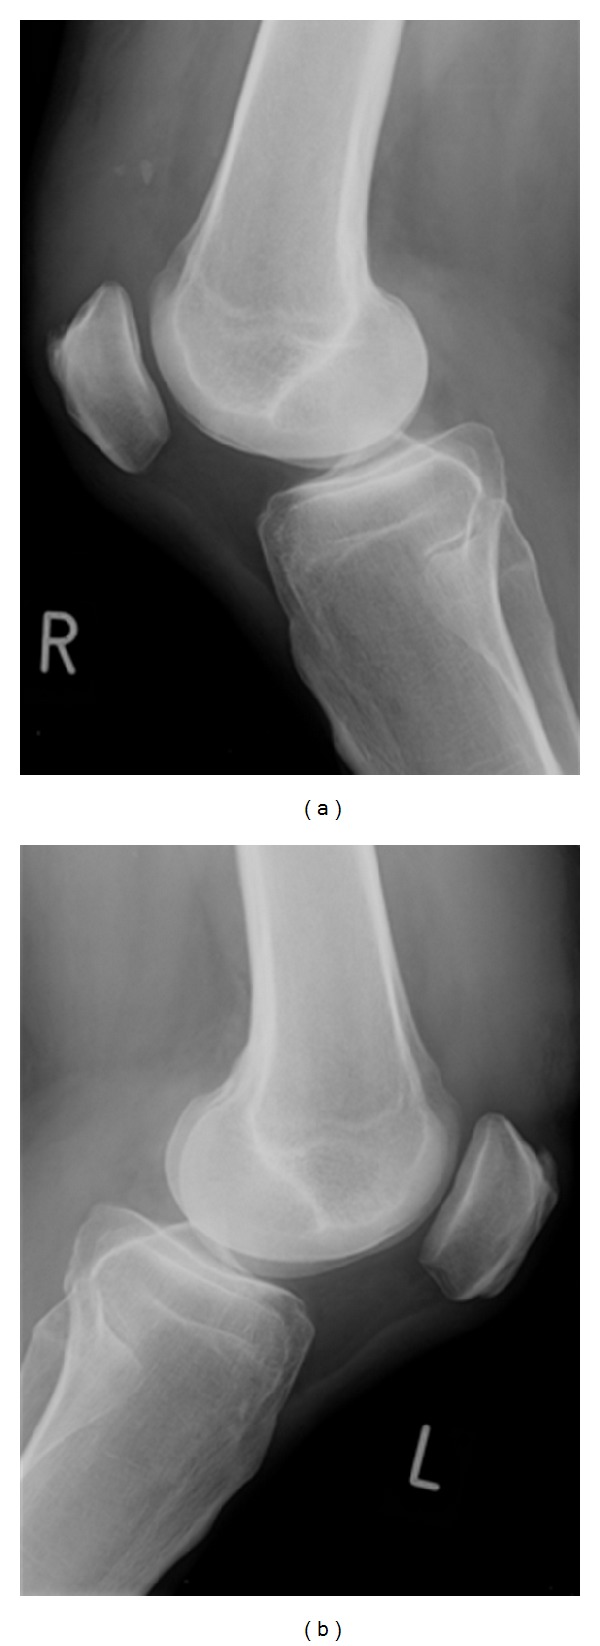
Plain radiographies show low riding of both patellae and signs of suprapatellar ossification of the right knee.
